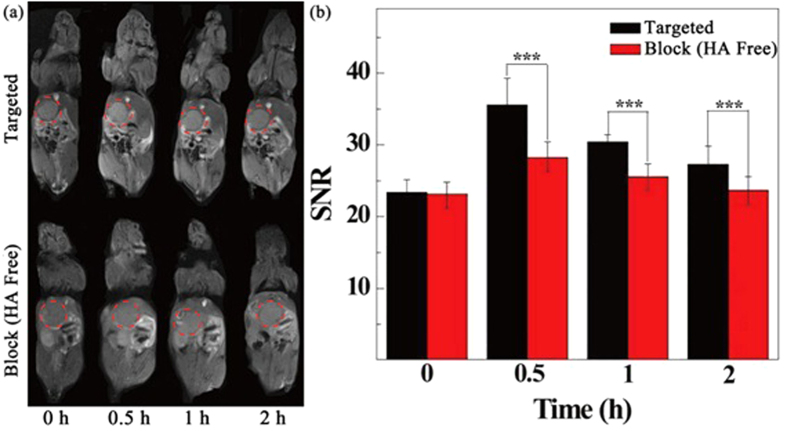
In vivo* MR images of orthotopic liver tumors at different times after an intravenous injection of 0.3 mL of a {(Au^0^)_100_G5.NH_2_-FI-DOTA(Mn)-HA} NP (300 μg Mn) solution in PBS.
